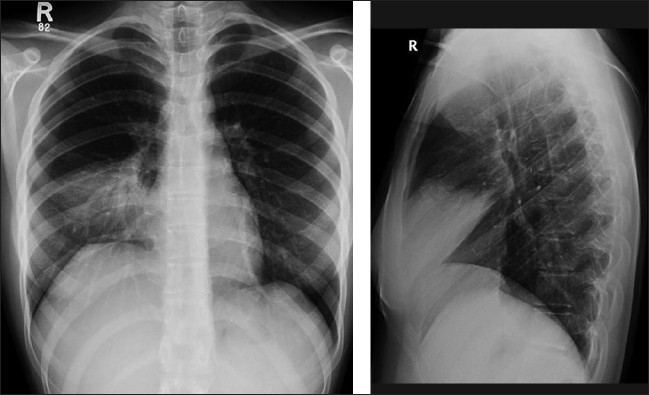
Right–middle-lobe atelectasis may cause minimal changes on an AP supine chest radiograph. Note the loss of definition of the right heart border. A collapsed right middle lobe is more clearly defined on lateral radiograph, which is not commonly available in the ICU patient. Attention to the fissures reveals that the horizontal and lower portions of the major fissures move towards each other resulting in a wedge of opacity pointing to the hilum. This is a middle-lobe consolidation mimicking middle-lobe atelectasis
